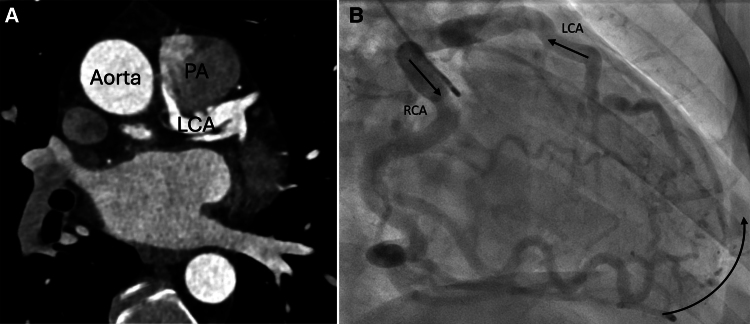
Adult-type ALCAPA: (*A*) CCTA shows the anomalous origin of the LCA from the PA instead of from the aorta. Contrast spillover from the LCA into the PA, as a pathophysiologic consequence of this variant. (*B*) ICA with contrast injection into the RCA shows pronounced collaterals and contrast spillover into the LCA (arrows). ALCAPA, anomalous left coronary artery from pulmonary artery; CCTA, coronary computed tomography angiography; LCA, left coronary artery; PA, pulmonary artery; RCA, right coronary artery; ICA, invasive coronary angiography.
